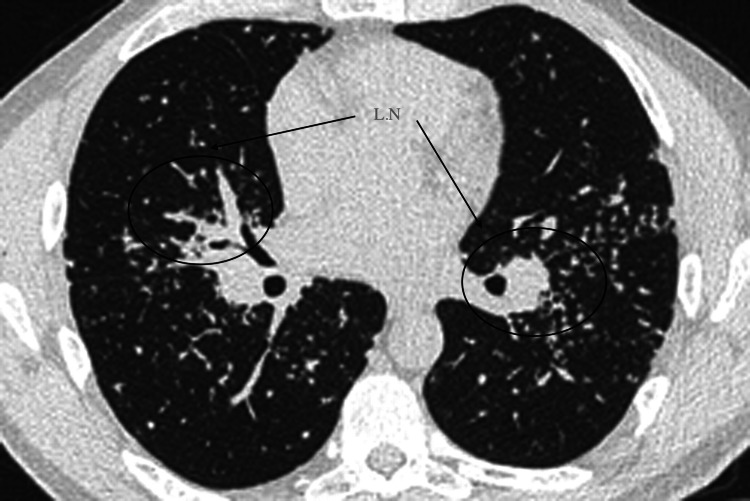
The CT scan of the chest revealed hilar lymphadenopathy, indicating the enlargement of lymph nodes in the pulmonary hilum. Lymph nodes were observed in the right upper lobe, interlobar region, middle lobe, and lower lobe, as well as in the left upper lobe, interlobar region, and lower lobe.
